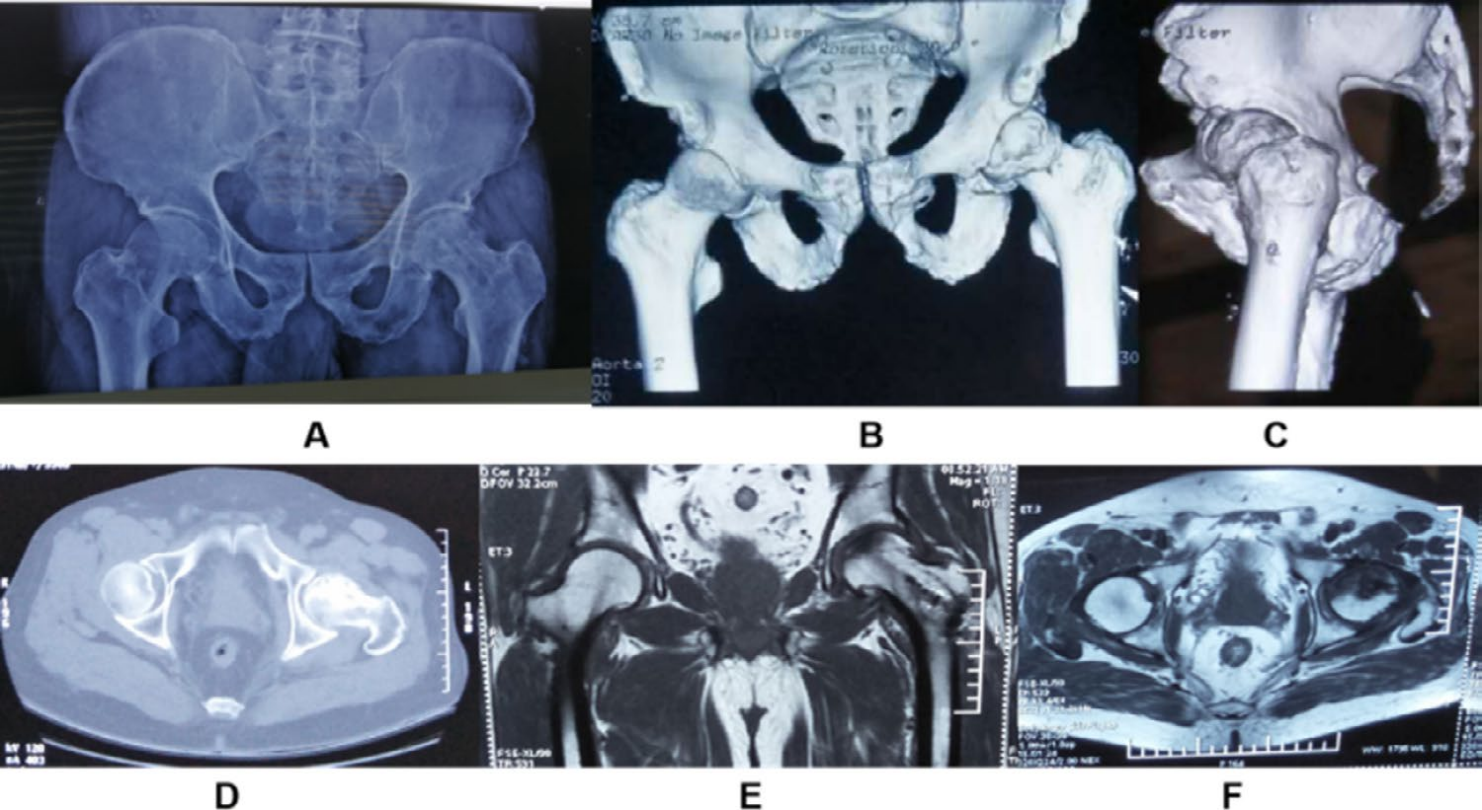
(A~F) Imaging performance at the 24 months postoperatively (bilateral) During follow-up, the patient did not see any significant narrowing and widening of the hip gaps bilaterally, and the X-ray showed bone filling in the capsular area. 2 years later, CT and MRI showed that the necrotic area was slowly replaced by some autologous bone, and the abnormal signal changes of bone and soft tissue were significantly restored compared with before, and the surface of the femoral head was less flat and did not collapse further, without pain and other There was no pain and other uncomfortable symptoms, and the patient was satisfied with the treatment:X-ray (A);CT (B,C,D);MRI (E,F)
